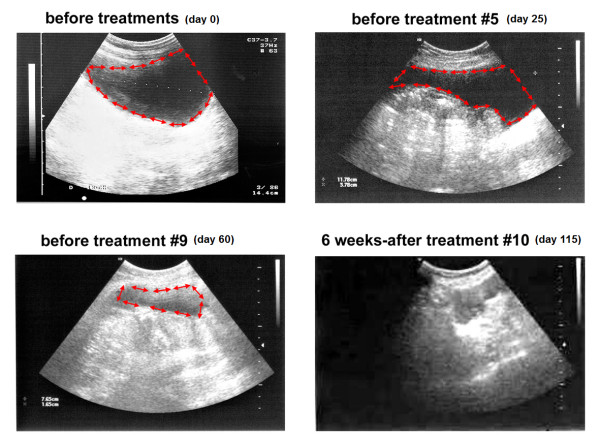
Ultrasound image of peritoneal cavity from compassionate patient with ovarian carcinoma treated with intra-peritoneal injection of the DTA-H19 plasmid. Ultrasound imaging was performed before each treatment to evaluate the amount of ascites fluid in our patient's peritoneum. A few representative figures of the imaging before the indicated treatments are presented. Red arrows indicate the limits around the area of ascites.
